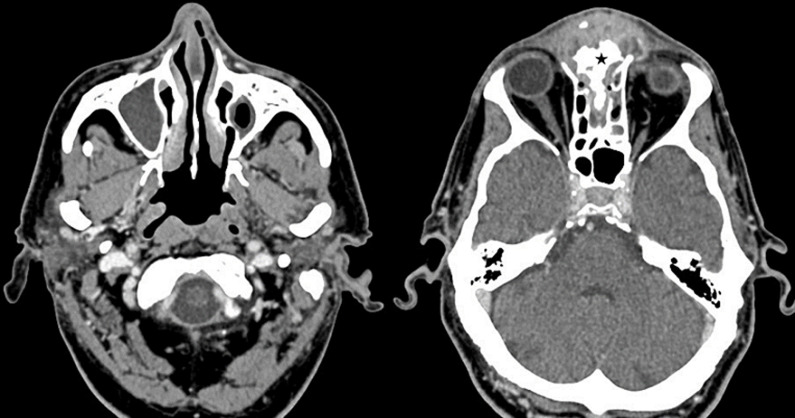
axial paranasal sinus computed tomography showing a pansinusitis with frontal osteitis and bone sequester (black asterix) surrounded by a soft tissue edema
